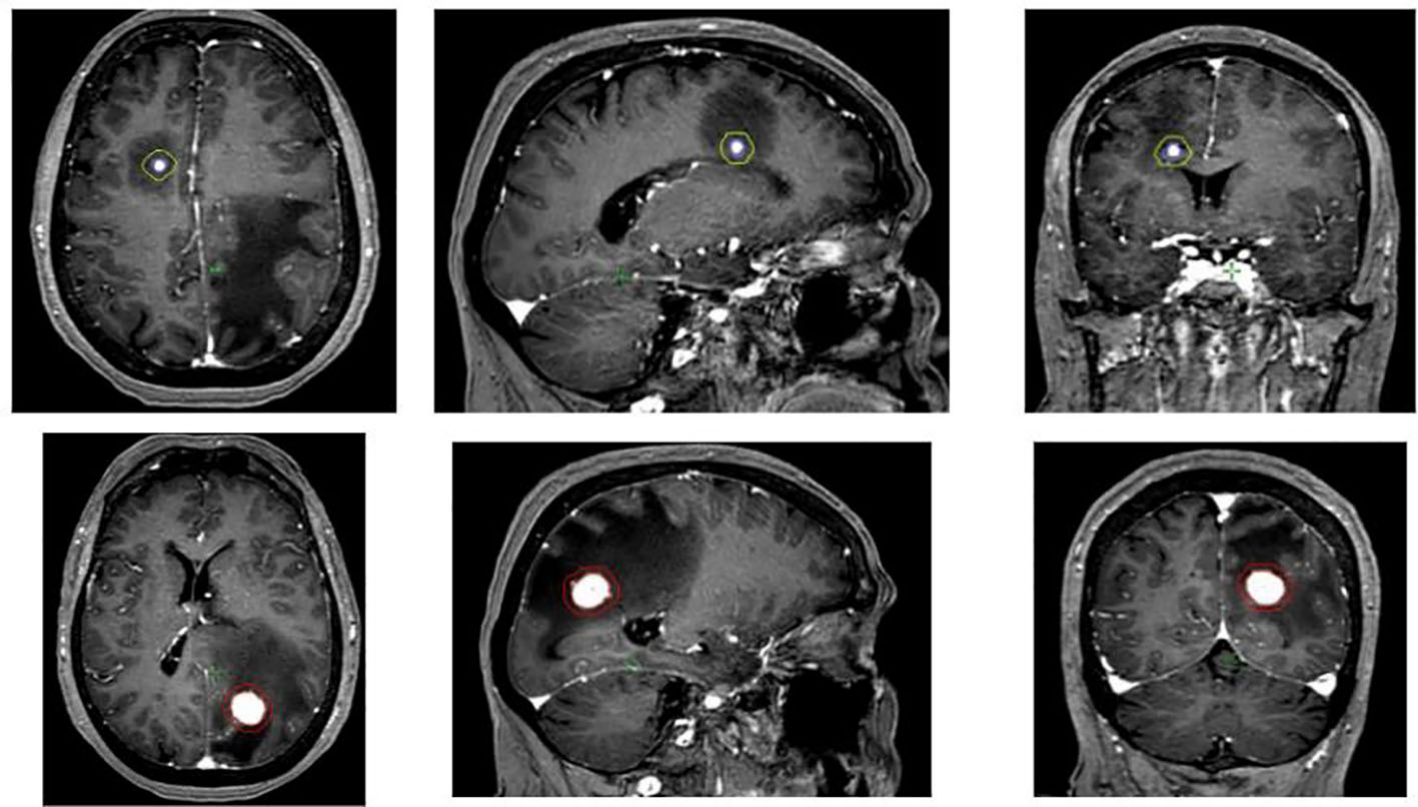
Brain MRI showing the two brain metastases, the right frontal (3 mm) and the left occipital one (18 mm). GTV was the T1-gadolinium enhanced lesion while PTV was defined as GTV + 3 mm.
